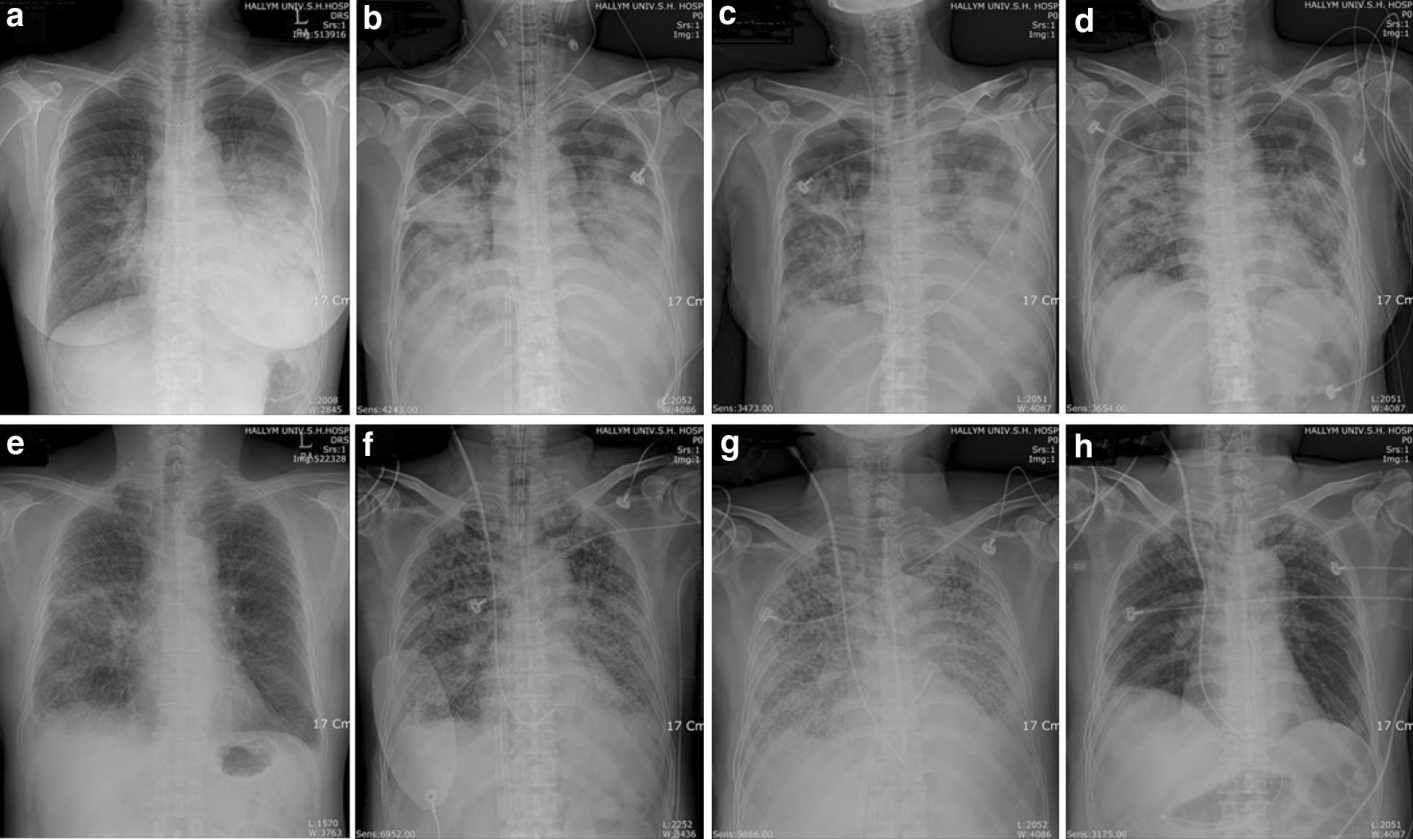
Chest radiography of two patients with severe ARDS caused by adenovirus. a and e On admission day; b and f on ECMO day 1; c and g on ECMO day 7 and; d and h on day after ECMO weaning
